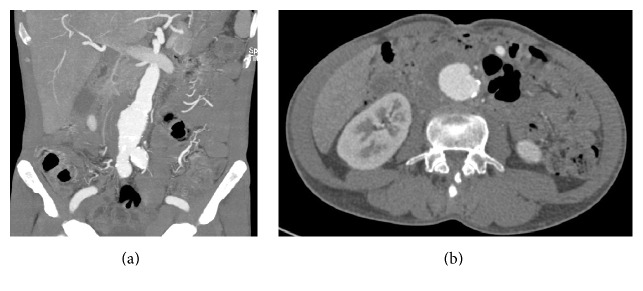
First patient: CTA abdomen. (a) Coronal section view of CTA: wall enhanced infrarenal AAA with periaortic tissue thickening and hematoma. (b) Cross-sectional view from CTA: blistering area of the aneurysm wall.
